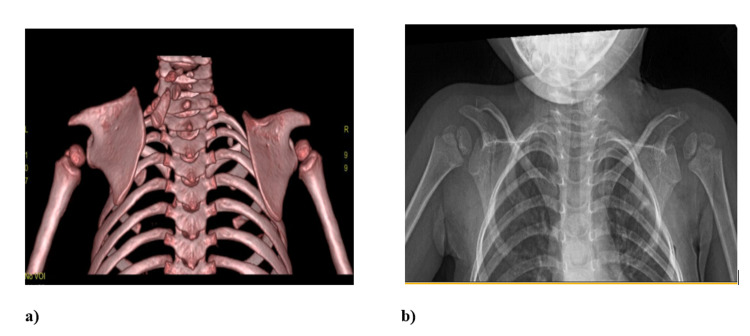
a) CT scan of a three-year-old patient with Sprengel's shoulder and omovertebral bone. b) Plain radiograph of the same three-year-old patient showing correction of Sprengel's deformity at follow-up post-Woodward’s procedure
